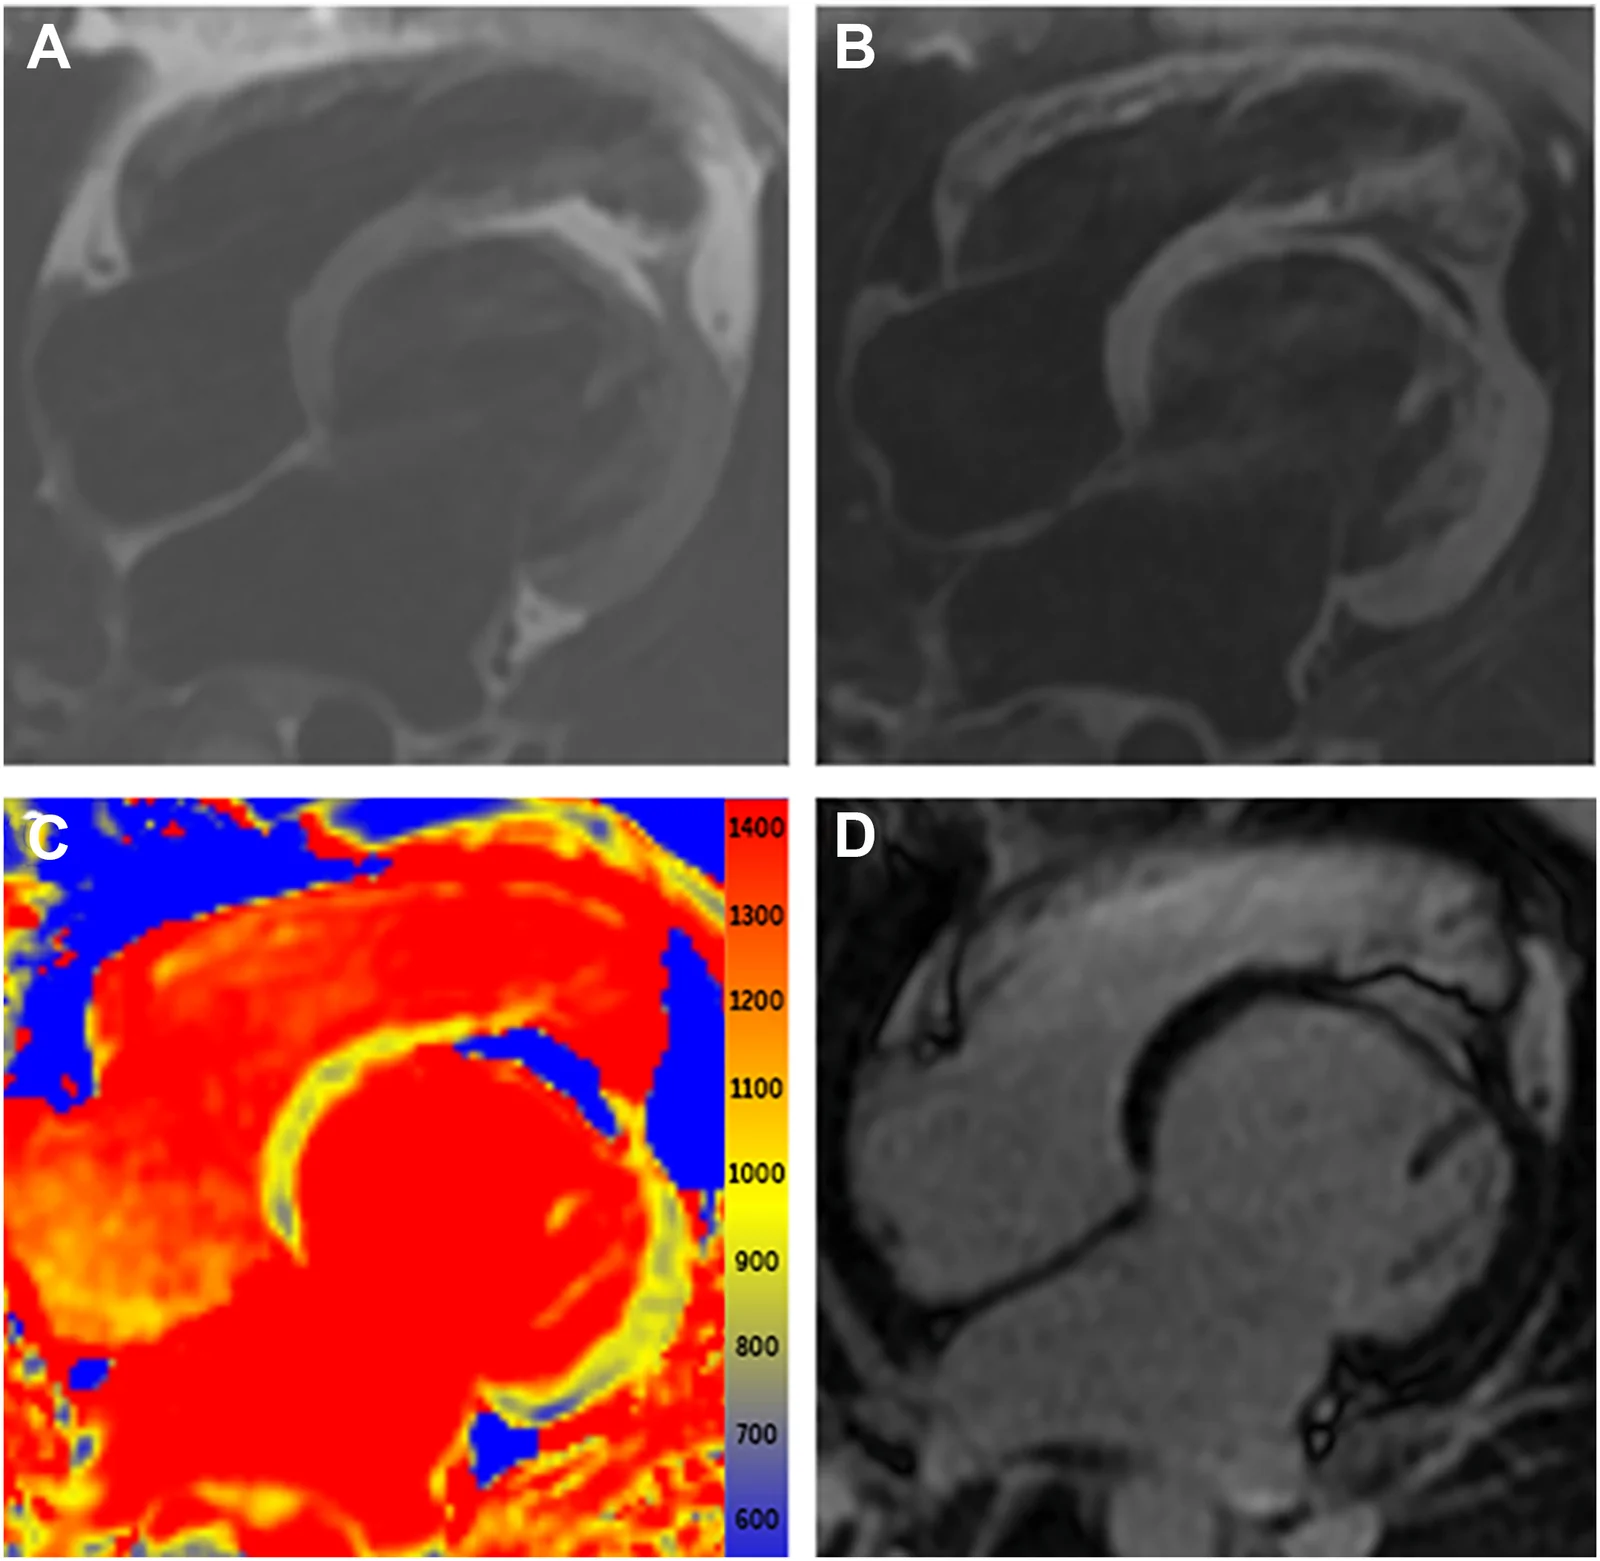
Cardiovascular Magnetic Resonance at Age 19 in Patient 2 With Tissue Characteristics Showing Apical Fatty Infiltration Without Myocardial Late Gadolinium Enhancement (A) T1-weighted image with turbo spin-echo showing a high apical signal suggestive of fatty infiltration. (B) T1-weighted imaging with fat saturation confirming apical fatty infiltration. (C) T1 mapping showing low native T1 values in the apex. (D) Four-chamber view showing no delayed enhancement.
